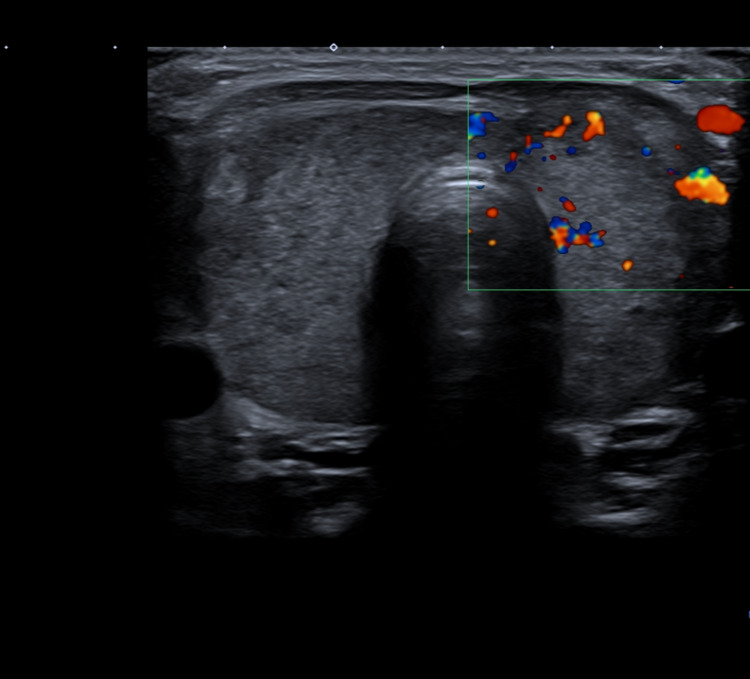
Thyroid ultrasonography. Thyroid ultrasonography showing an enlarged gland with heterogeneous parenchyma with associated hypervascularity, suggesting thyroiditis.
